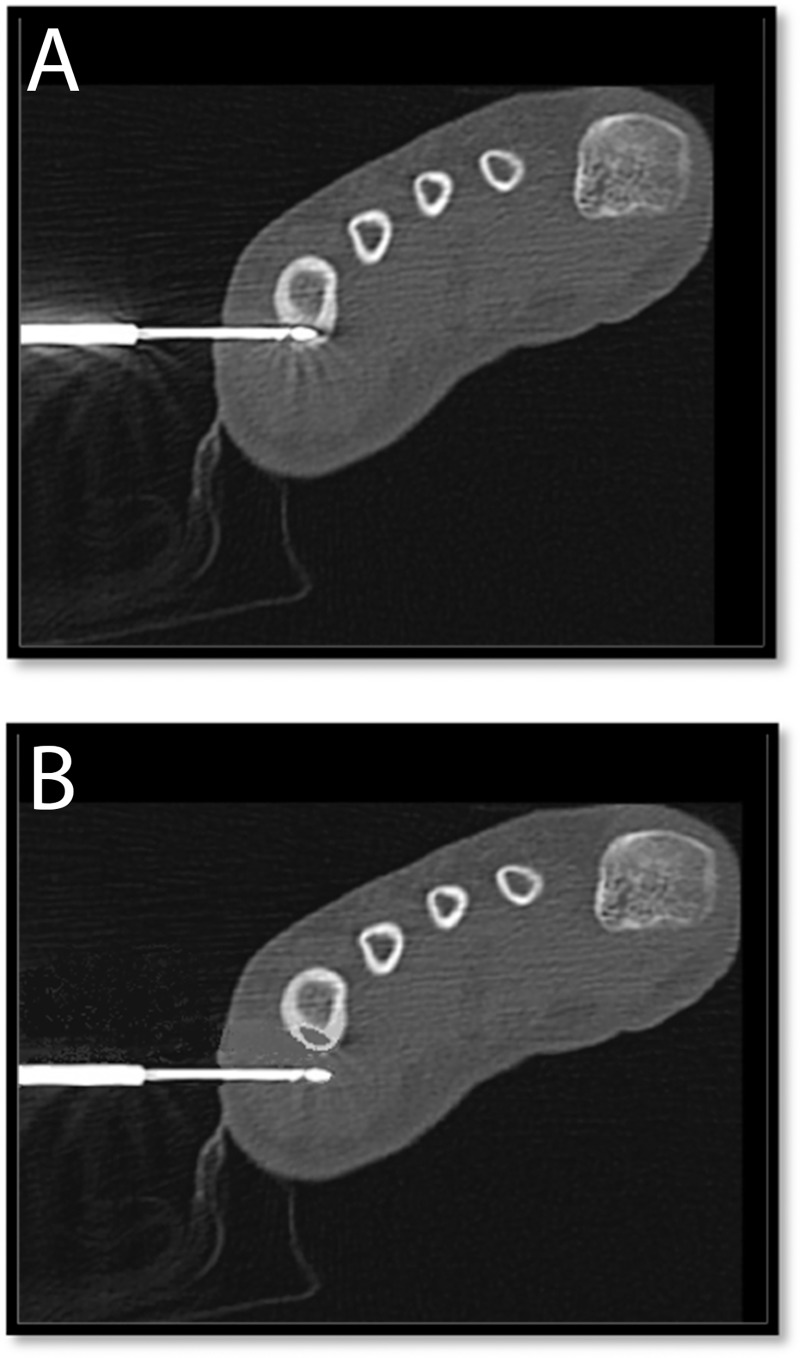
CT images of an ablation needle in situ. Osteoid osteoma in 5th metatarsal. A: Needle in nidus (accurate placement). B: Needle outside nidus (= inaccurate placement).
